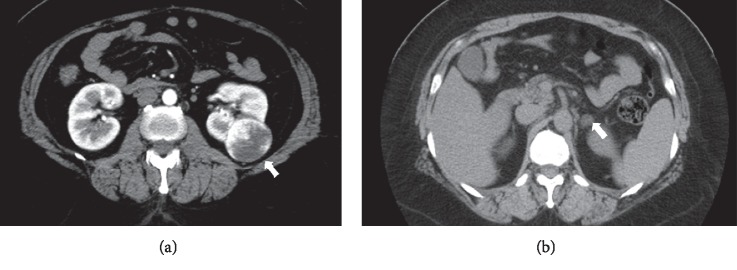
The computed tomographic (CT) findings of the left adrenal tumor. (a) Renal tumor in the arterial phase. Most of the left renal tumor was enhanced using contrast medium, except the necrotic area. (b) Adrenal tumor. The left adrenal tumor was a low-density mass having a diameter of 10 mm and was suspected as adrenocortical adenoma.
